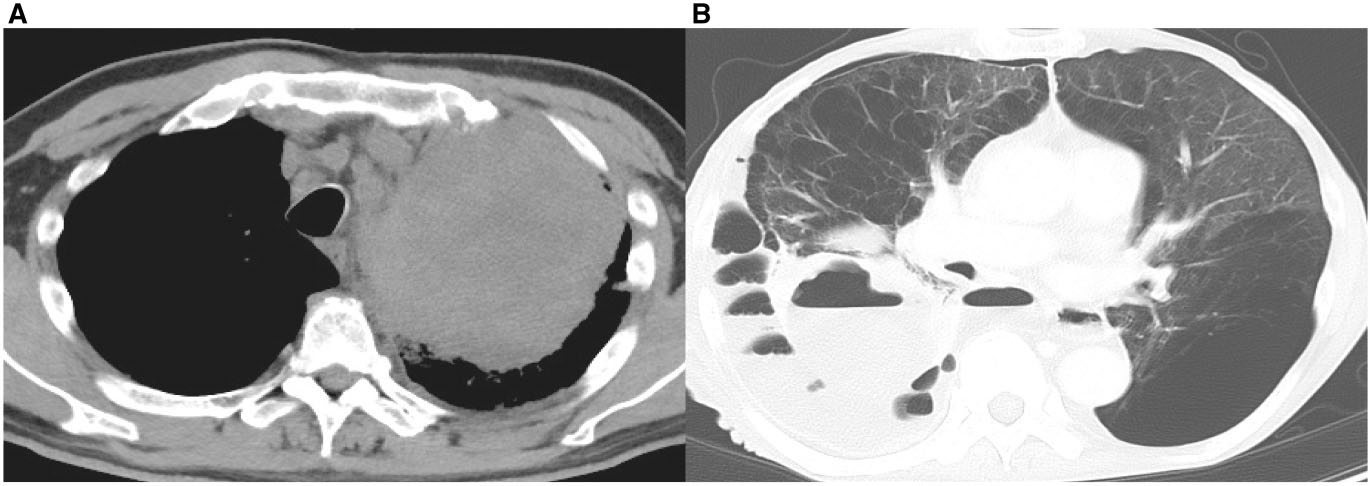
(A) Preoperative computed tomography of patient number 5 diagnosed with a huge pleomorphic carcinoma located in the left upper lobe. The tumour changed into a necrotic mass after chemotherapy. (B) Preoperative computed tomography of patient number 9 diagnosed with a large squamous cell carcinoma located in the right lower lobe. The tumour was broken and complicated with acute empyema after chemotherapy.
